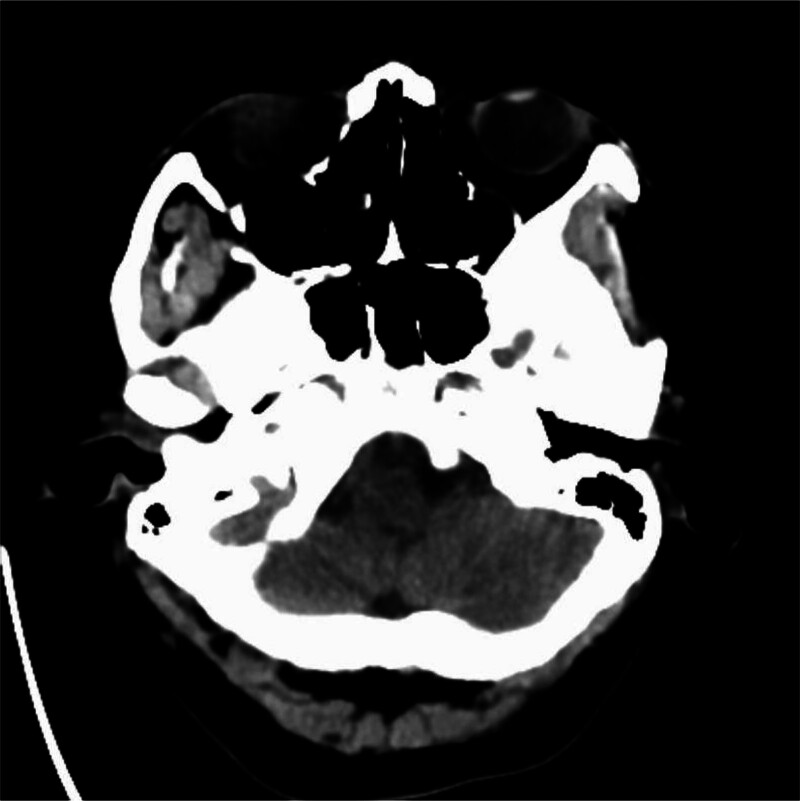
On January 24, 2019, non-contrast CT images of the patient.
